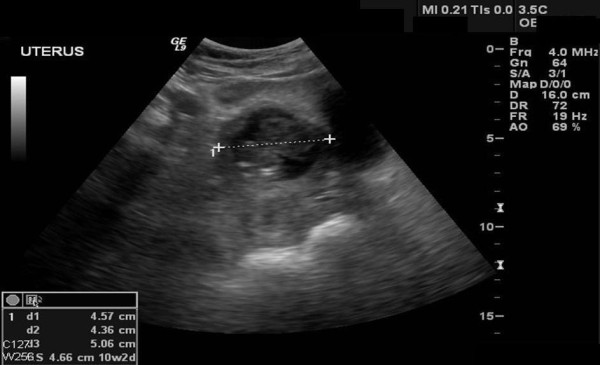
Abdominal ultrasound, transverse scan showing a gestational sac measuring 4.57 × 4.36 cm between the uterus and the urinary bladder.
